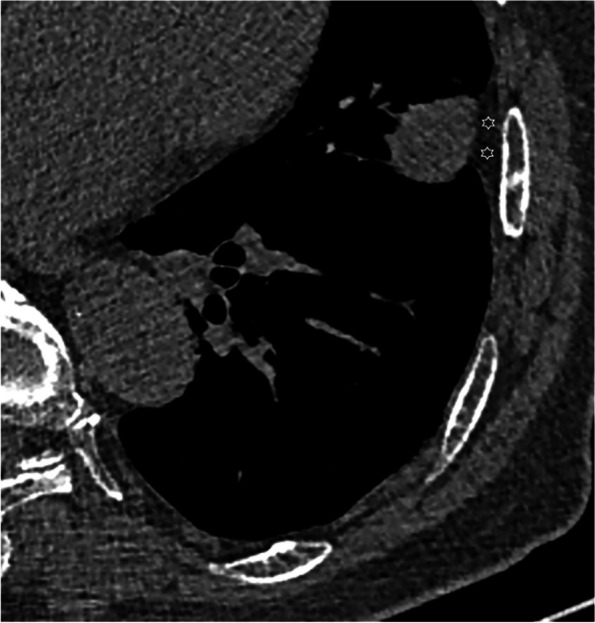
SPAN with extrapleural fat thickening. A 76-year-old man with left upper lobe SPAN which is confirmed as invasive adenocarcinoma. On the axial CT image, significantly thickened extrapleural fat (asterisks) protrudes into the lung field
